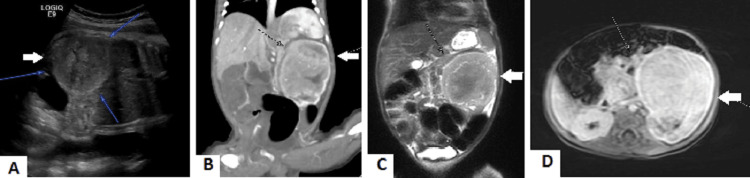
Enlarged left kidney showing a well-defined mass lesion with homogeneous signals and enhancement. (A) Intrauterine fetal ultrasound. (B) Coronal CT of the abdomen. (C) Coronal T2-weighted MRI. (D) Axial MRI.
